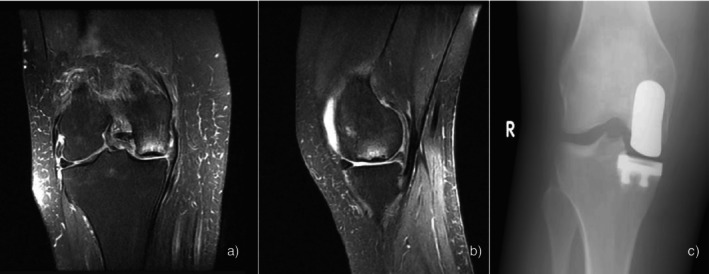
(a) Coronal and (b) sagittal T2‐weighted magnetic resonance images showing a focal subchondral patch of increased bone marrow signal of the medial femoral condyle. (c) Anteroposterior radiograph showing the knee after UNI.
